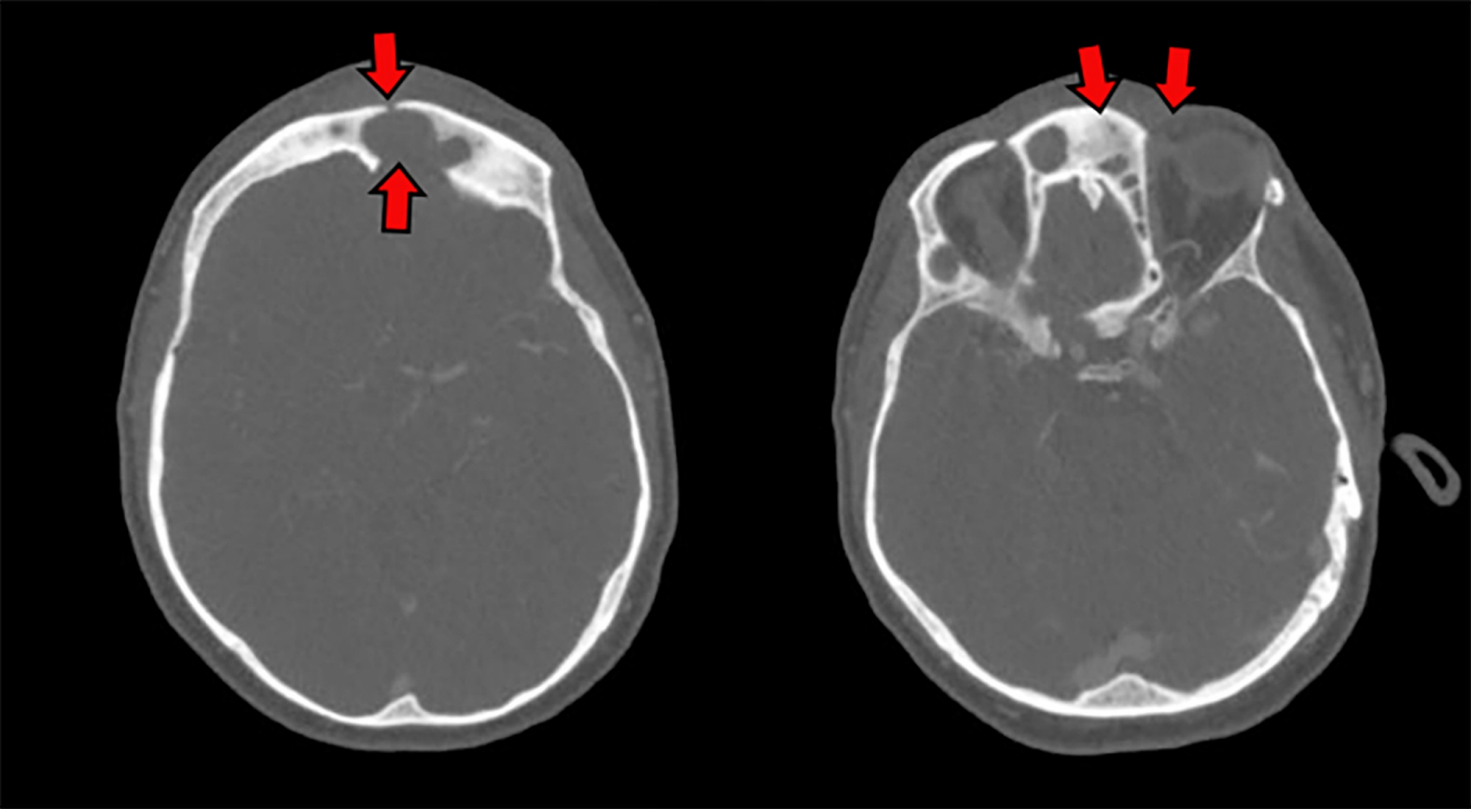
CT scan (Case 2) Contrast-enhanced axial computed tomography (CT) scan showing a large bony erosion of the internal wall of the left frontal sinus and a small bony erosion of the anterior wall (red arrows).
